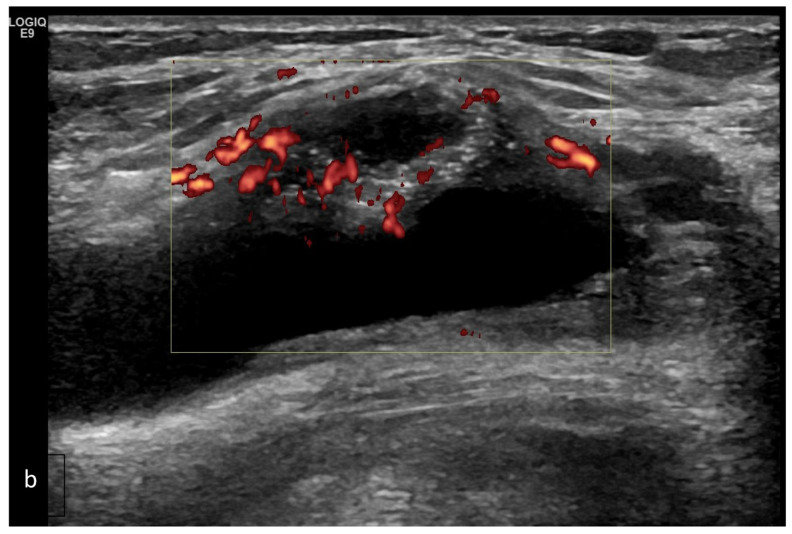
Posterior longitudinal US scan of the knee joint for the assessment of the popliteal fossa of a patient with knee OA. (a) Baker’s cyst with effusion (*), where the heterogeneous abnormal hypo-/hyperechoic mass is consistent with synovitis (blue arrows). Hyperechoic spots are an indicator of crystal deposition (orange arrows). (b) The same patient from (a). Power Doppler US modality reveals synovitis with a high degree of activity.
